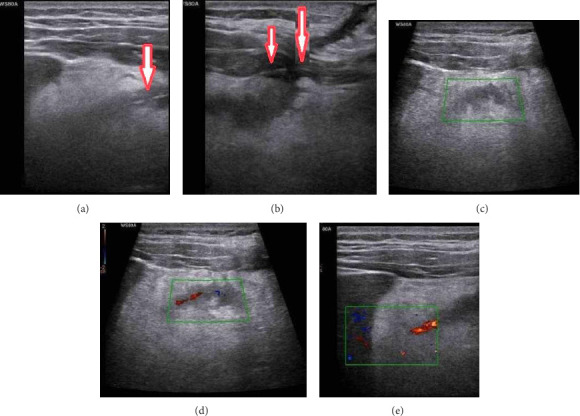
Gray-scale ultrasonography revealed a hyperechoic line at the superficial aspect of the catheter track, with no evidence of the suture path at deeper levels (a-b, red arrows). Color Doppler ultrasound was then used. Although the color Doppler was active, no signal was observed as the suture had not been manipulated (c). After manipulating the suture, a linear Doppler signal was observed, corresponding to the path of the manipulated suture (d-e). The pulse repetition frequency and Doppler settings used were G50/0.40 kHz/F1/FA8, with the flow velocity estimated to be within the expected physiological range for superficial soft tissue perfusion.
